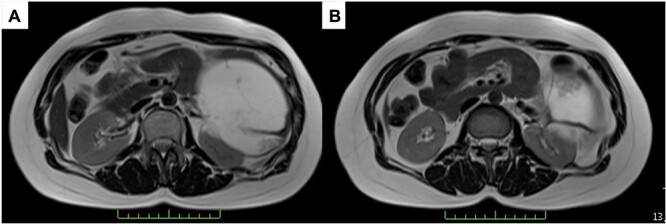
T2-weighted MRI; (A) the tumor has a thick fibrous capsule; (B) the tumor has a thick fibrous septum.
